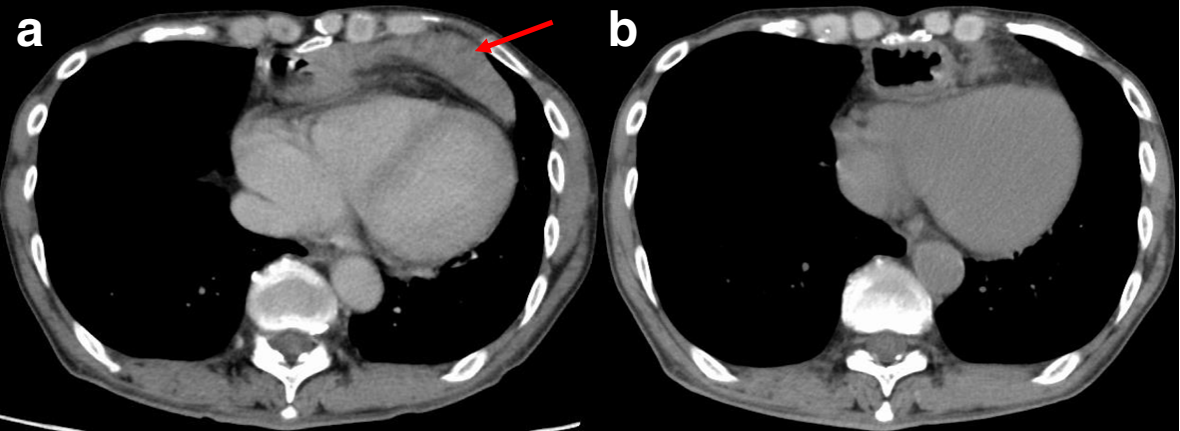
Computed tomography findings in case 2. a Computed tomography image obtained on the day of symptom onset. The intestine (red arrow) was dislocated only in the retrosternal space. b Computed tomography image obtained 2 days after symptom onset. The intestine had disappeared from the retrosternal space
